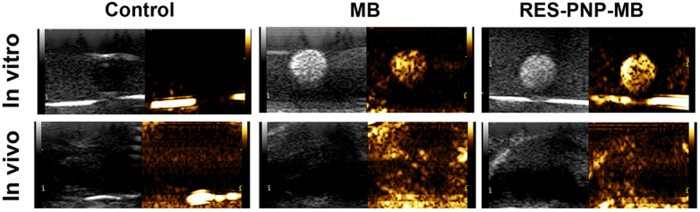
In vitro* and *in vivo* ultrasound images of degassed and deionized water (control), microbubbles, and RES-PNP-MB.
